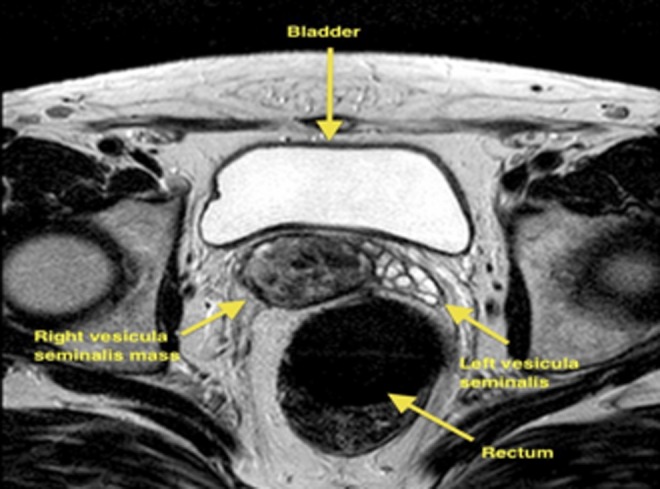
MRI demonstrating a right seminal vesicular mass between the bladder and rectum.
